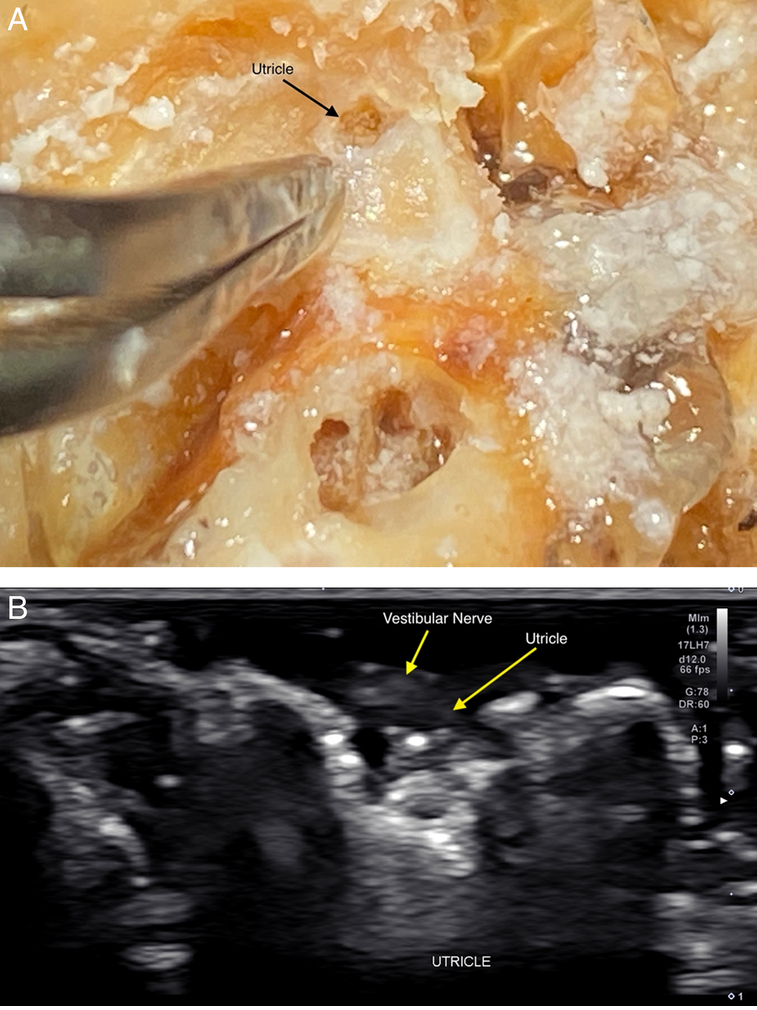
External appearance of the utricle with the vestibular nerve removed (A). Sonogram (17 MHz) demonstrating the identification of the utricle location and overlying short-axis view of a branch of the vestibular nerve (B).
